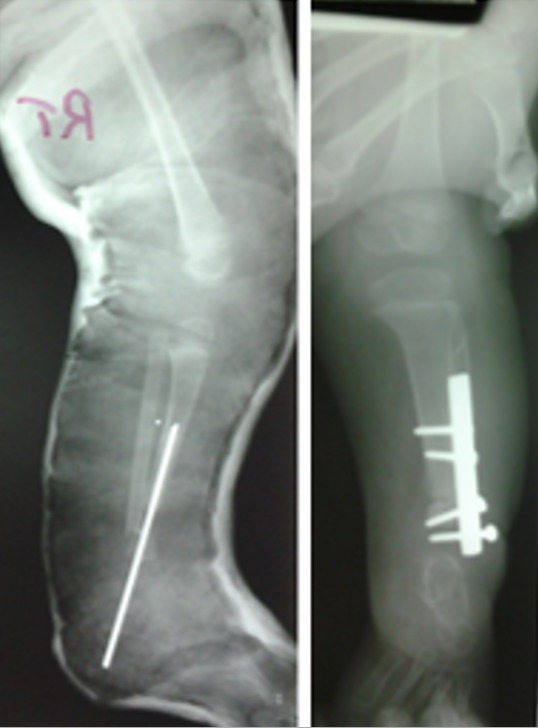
Radiographs after tibiofibular synostosis and rash rod insertion and after exchange to plating
